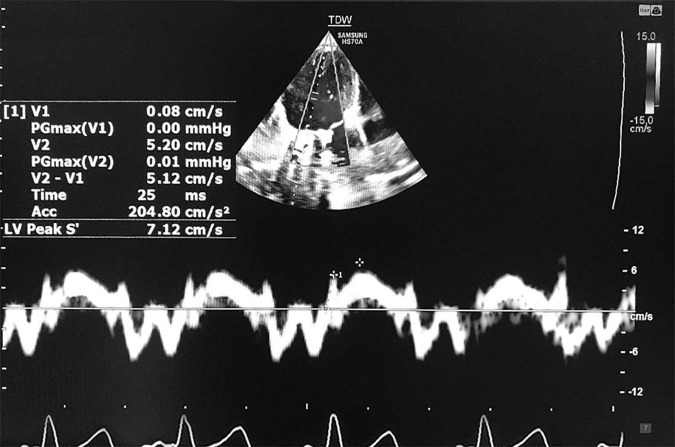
Measurement of tissue Doppler-derived IVA and s’ velocity. IVA, myocardial acceleration during isovolumic contraction; s′, systolic longitudinal myocardial velocity.
